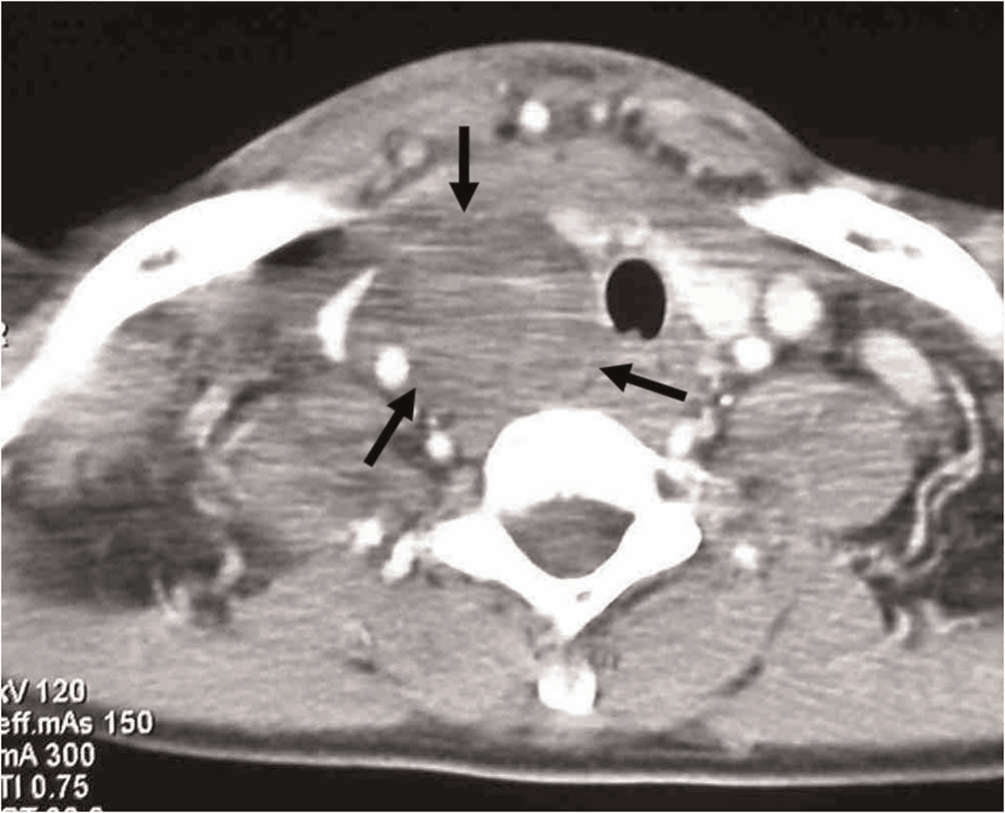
Computed tomography scan on admission (at thoracic inlet level) showing signs of cervical-mediastinal hematoma (black arrows) in the right prevertebral and paratracheal space. A marked midline shifting and compression of the trachea is evident.
